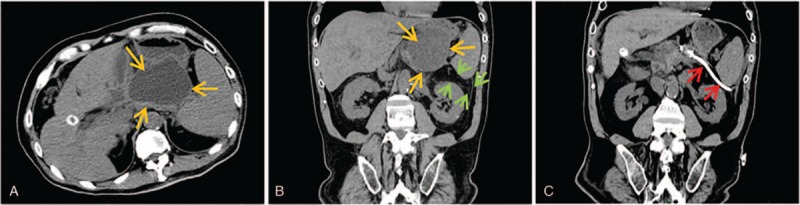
MPR-assisted pararenal space approach. (A) Axial CT scans showed an abscess (light orange arrow) with size of 7 × 8 cm was detected on top of pancreatic body and tail and surrounded by spleen and stomach. (B) MPR technique showed that a cross-sectional entrance along interstitial space of spleen and kidney (green arrow) into the abscess cavity (light orange arrow). (C) CT scans obtained 1 week after the procedure showed that drainage catheter (red arrow) advanced along the proposed access route and the abscess cavity collapsed.
